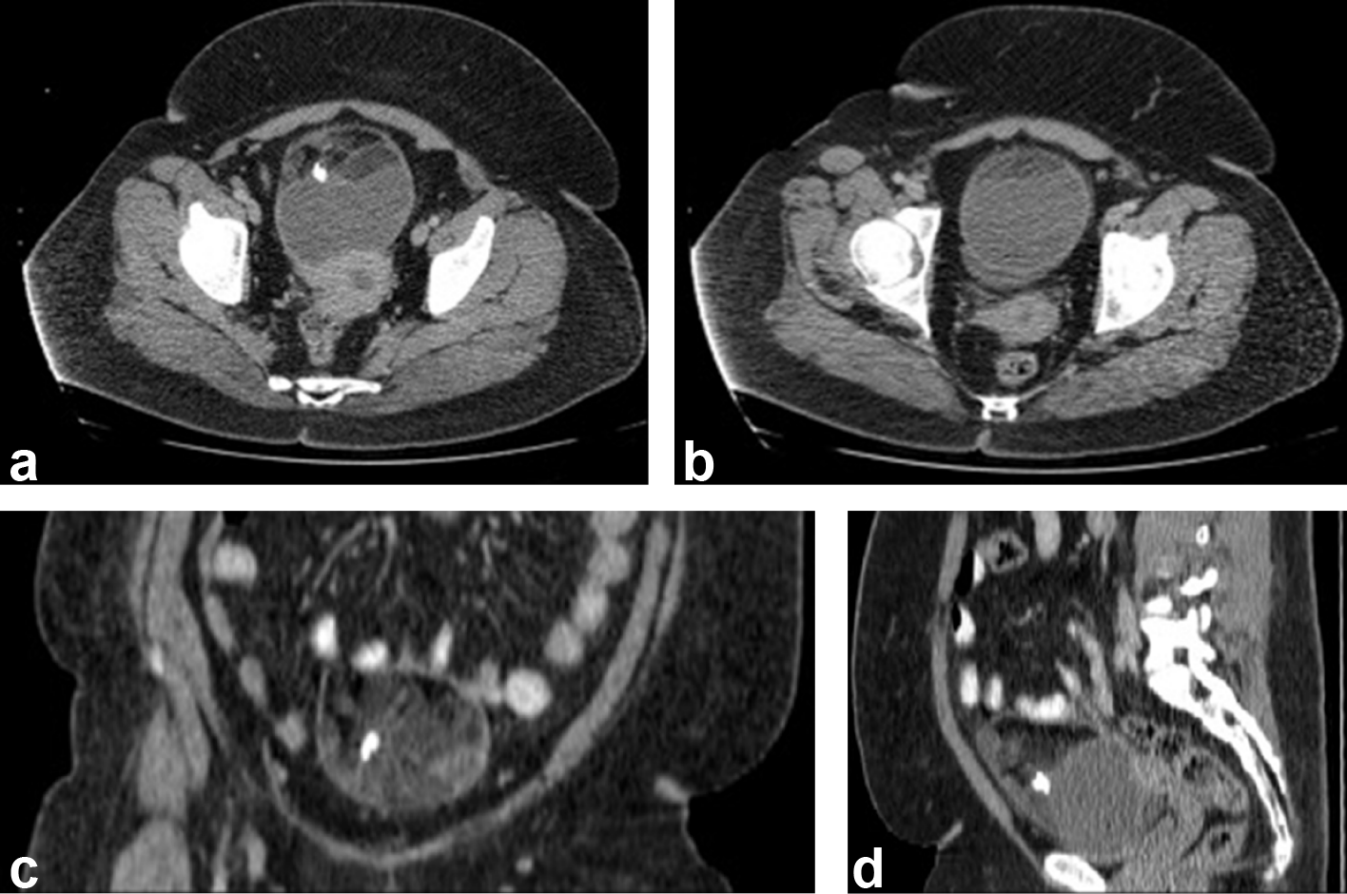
(a, b) Axial, (c) coronal and (d) sagittal. These CT abdomen image 5 years prior shows a 10 cm ovarian mature benign cystic teratoma.
